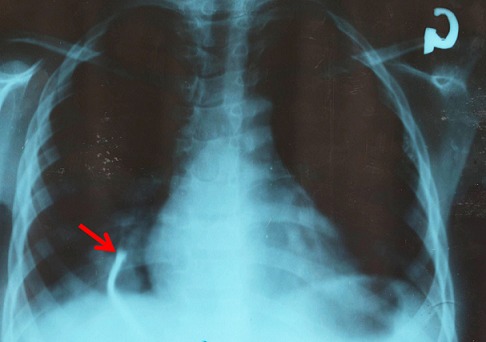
Radiographie du thorax vue de face montrant la progression du clou dans la bronche droite vers le bas 36 mois après son inhalation
